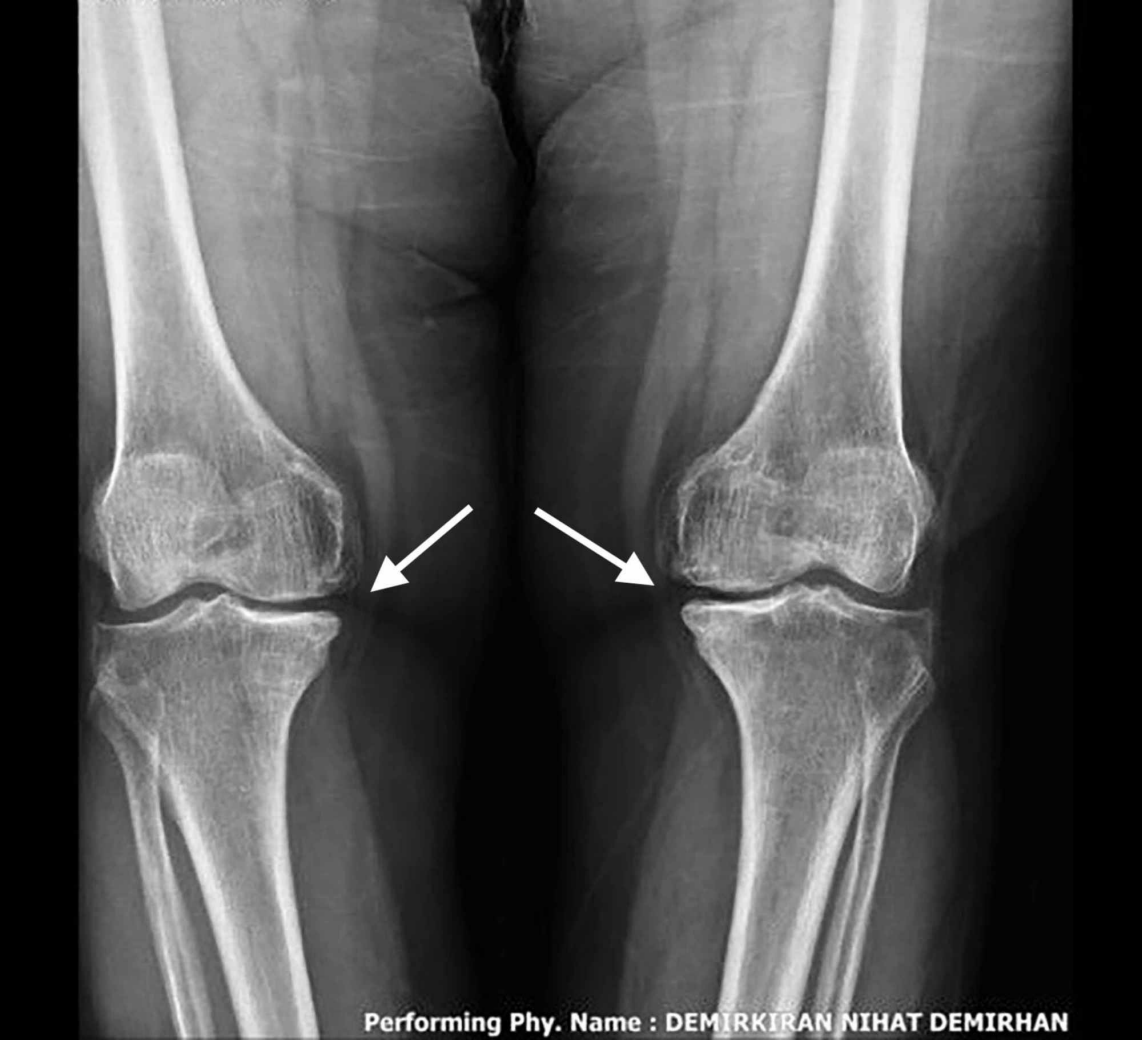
Preoperative antero-posterior (AP) radiograph of both knees; arrows indicate the medial joint space narrowing and osteophyte formation
